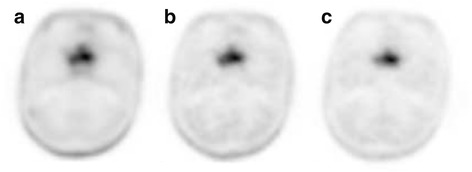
Representative ^18^F–BPA PET images of malignant melanoma in a 39-year-old woman with sphenoid sinus melanoma. ^18^F–BPA PET images at (a) 30 min (SUVmax = 8.2, TNR = 7.5, TBR = 5.9), (b) 60 min (SUVmax = 9.6, TNR = 9.6, TBR = 6.9), and (c) 120 min (SUVmax = 9.3, TNR = 9.3, TBR = 7.2) after injection
